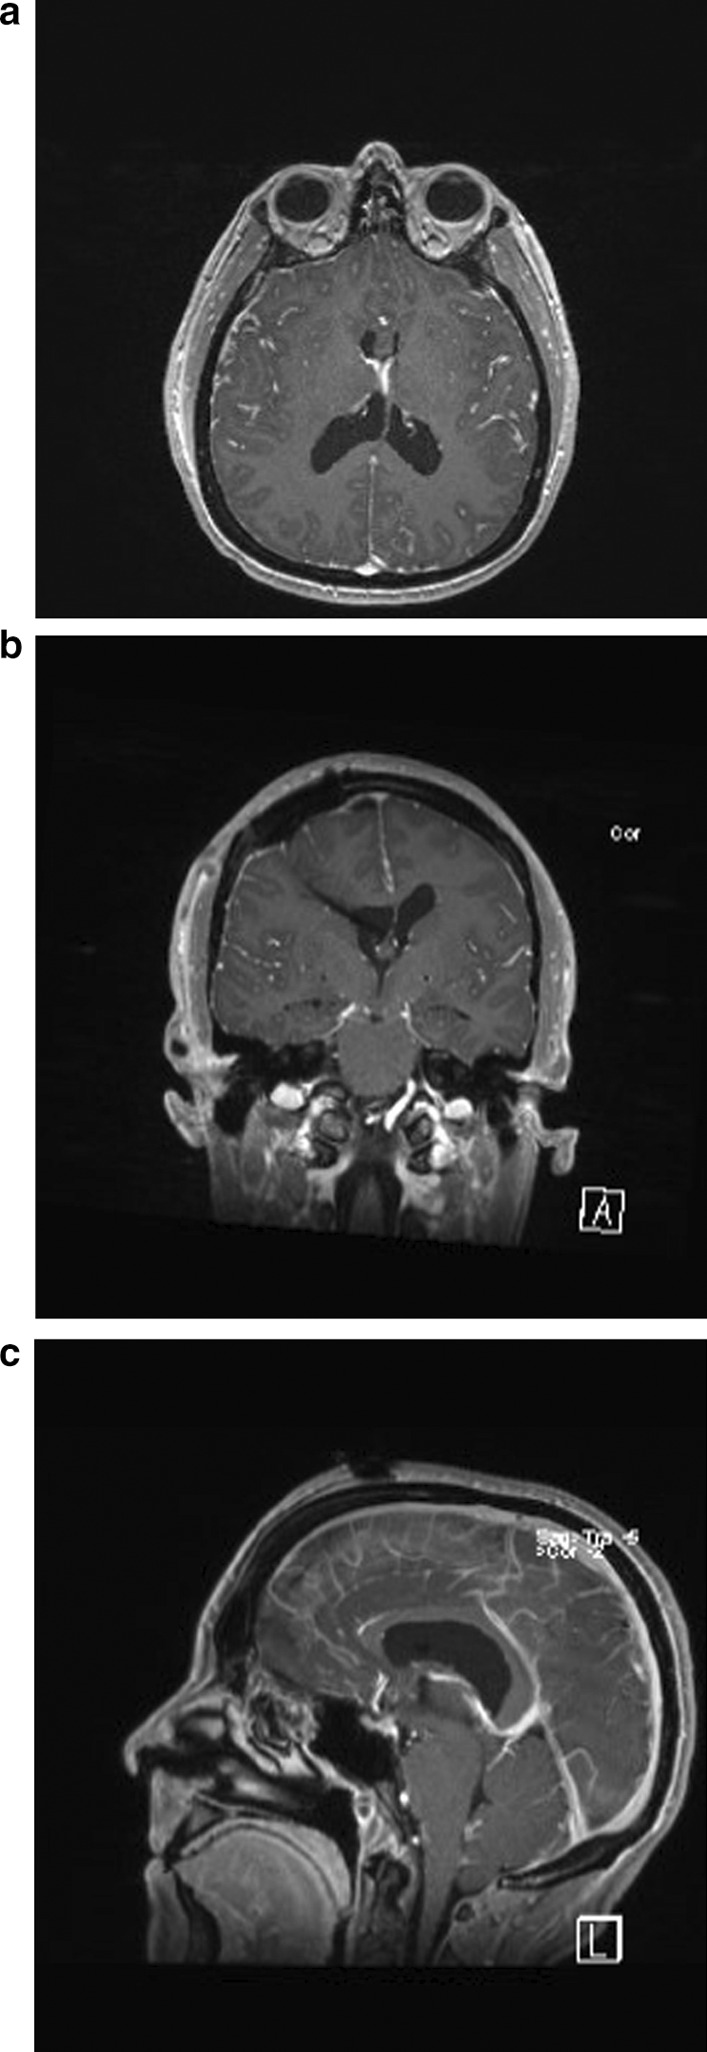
Postoperative axial (a), coronal (b), and sagittal (c) T1 postcontrast magnetic resonance imaging demonstrating complete resection of the cyst with mild ventriculomegaly and small amount of intraventricular hemorrhage
